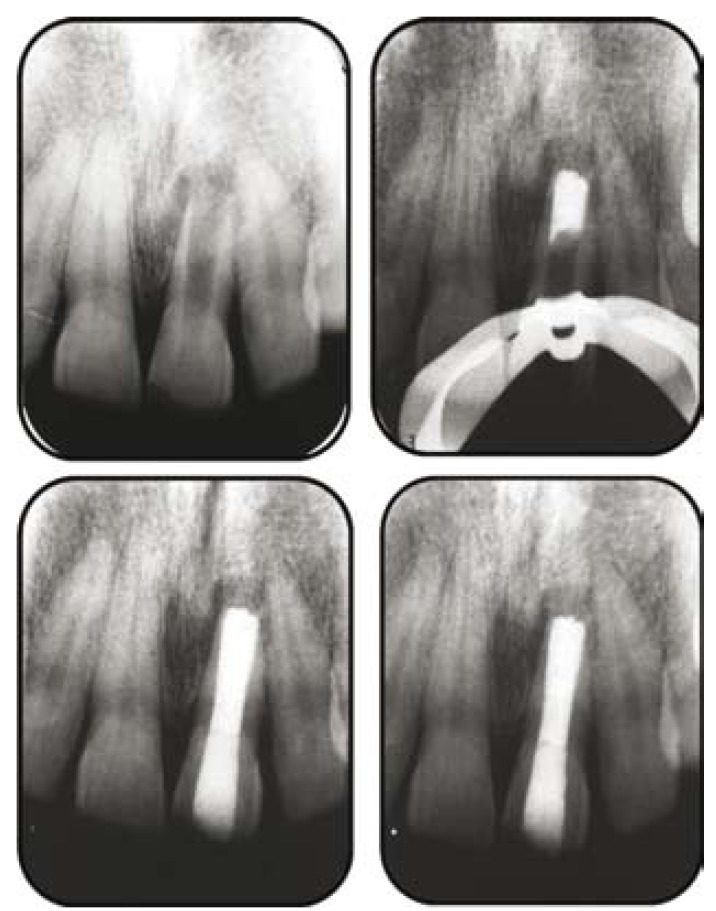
a) Diagnostic radiograph; b) MTA plug; c) Post obturation radiograph; d) Healing evident after 3 months.
